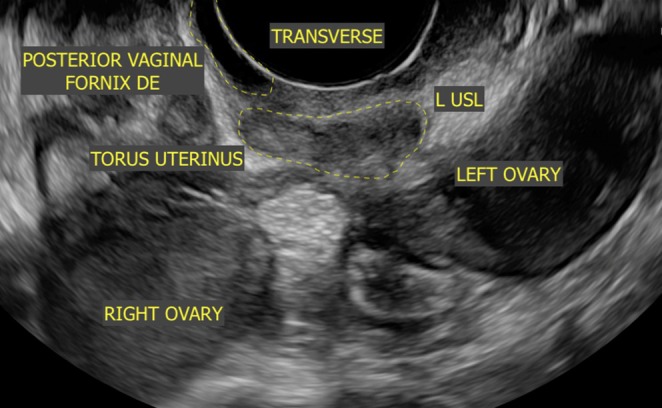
Sonographic characterisation of DE of the USLs and TU in the presence of POD obliteration in transverse. DE, deep endometriosis, LUSL, left uterosacral ligament; TU, torus uterinus.
